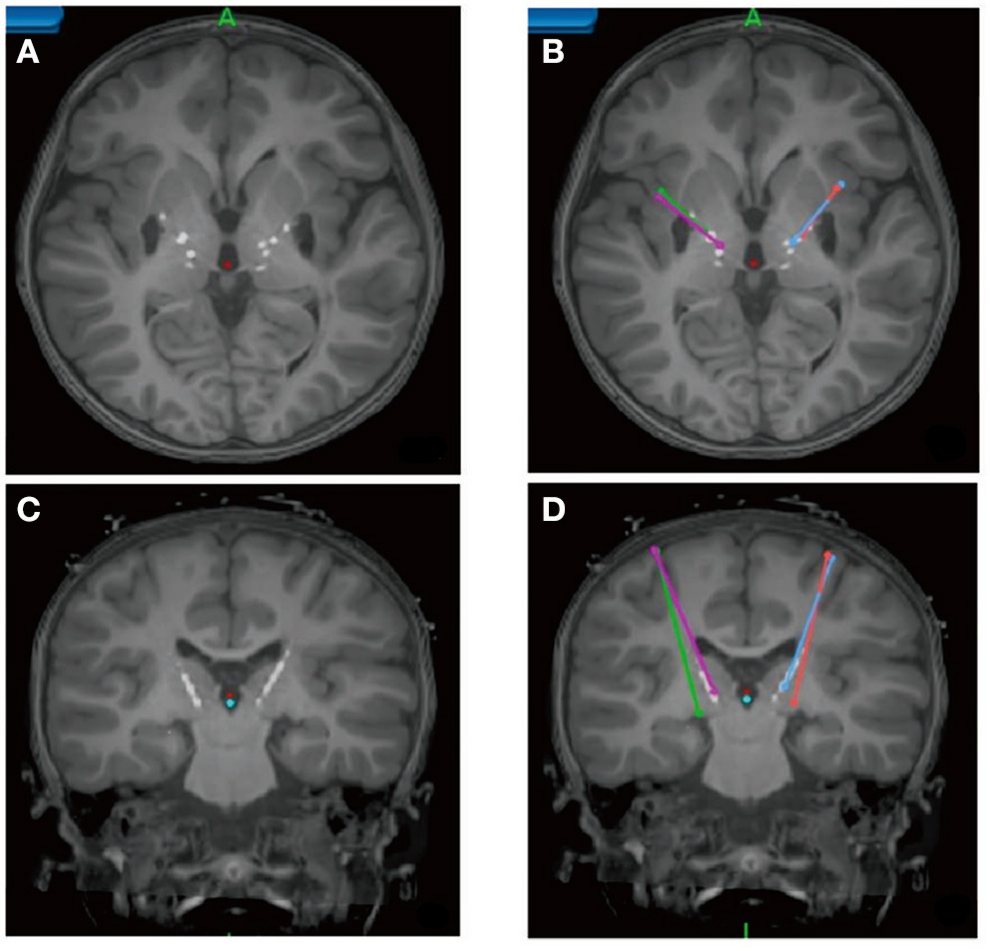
Pediatric Deep Brain Stimulation Using Awake Recording and Stimulation for Target Selection in an Inpatient Neuromodulation Monitoring Unit (170). Axial (A,B) and coronal (C,D) MRI showing the position of temporary leads within the basal ganglia and thalamus. Stereotactic planning trajectories are shown in (B,D).
